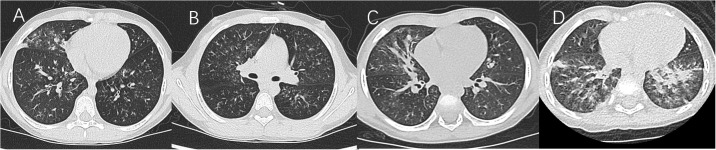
(A, B) Case 1. (C) Lung HRCT showed unilateral/bilateral inflammatory bronchiolitis including typical diffuse tree-in-bud signs and centrilobular nodules. (D) Lung HRCT showed diffuse high-density ground-glass opacification in bilateral lungs.
